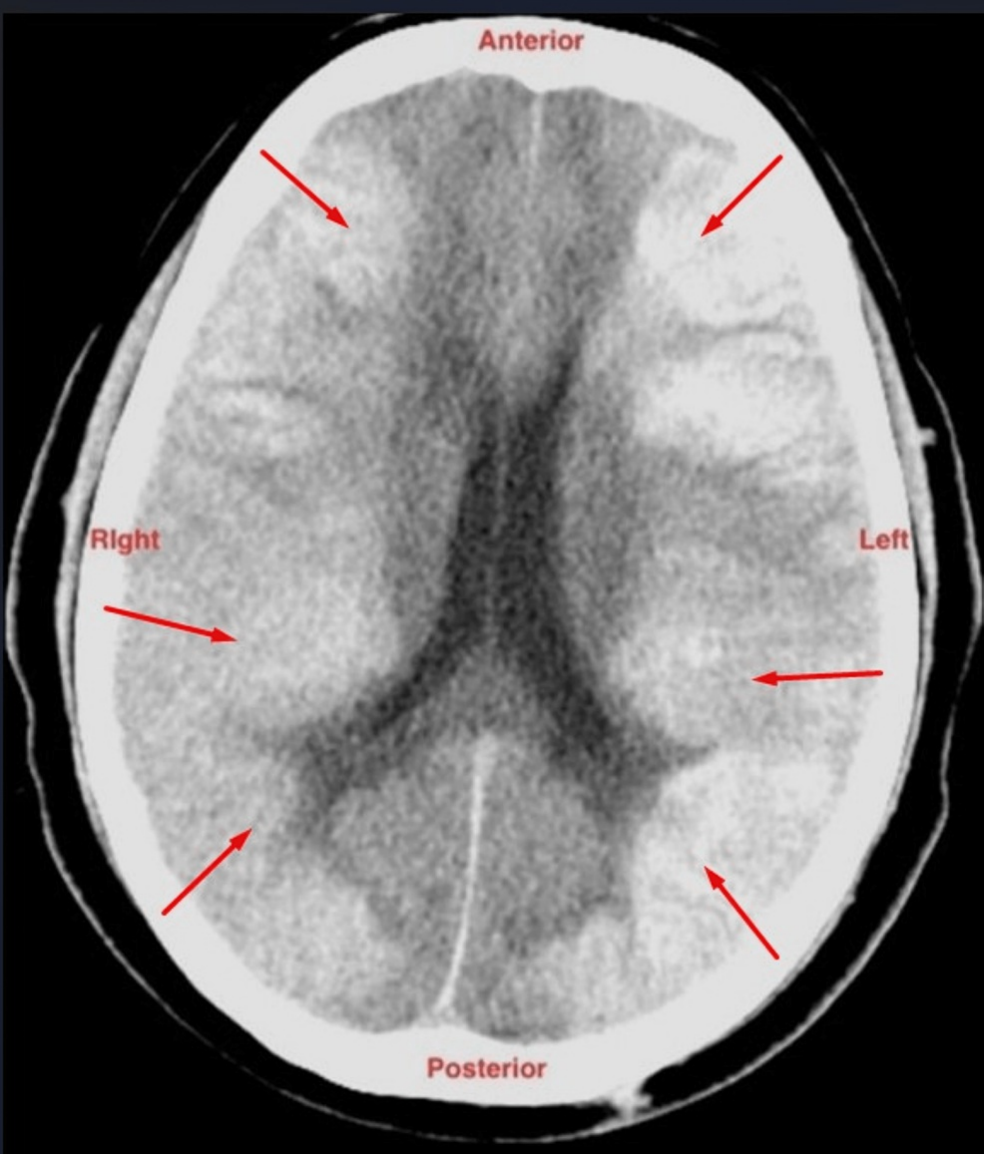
CT scan of the head revealing the presence of a bilateral subarachnoid hemorrhage. CT, computed tomography
